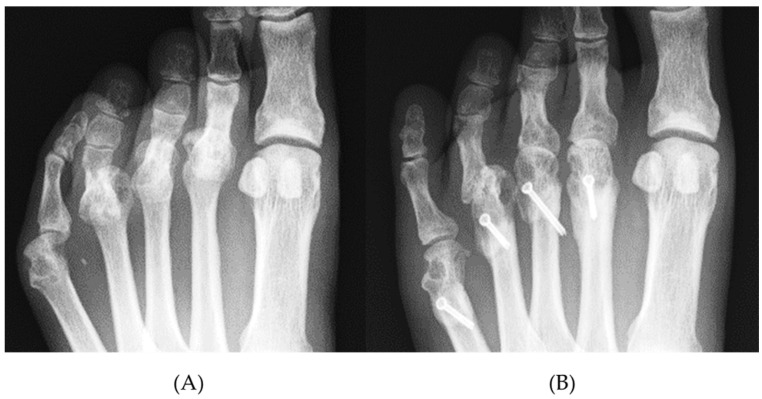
Radiographic findings before (A) and after (B) shortening oblique osteotomy for lesser metatarsal bones. The preoperative MOD of each toe was 15.9 mm (grade 3), 18.9 mm (grade 3), 17.4 mm (grade 3), and 5.5 mm (grade 2) in the 2nd–5th toes, respectively. Postoperative MOD grades were ameliorated to grade 1 in the 2nd, 3rd, 5th MTP joint. In the 4th MTP joint, postoperative MOD was 10.9 mm (grade 3), and there was a recurrent dorsal dislocation, causing the remnant callosity on the plantar side. The ratio of metatarsal shortening/preoperative MOD (%) was 30%.
